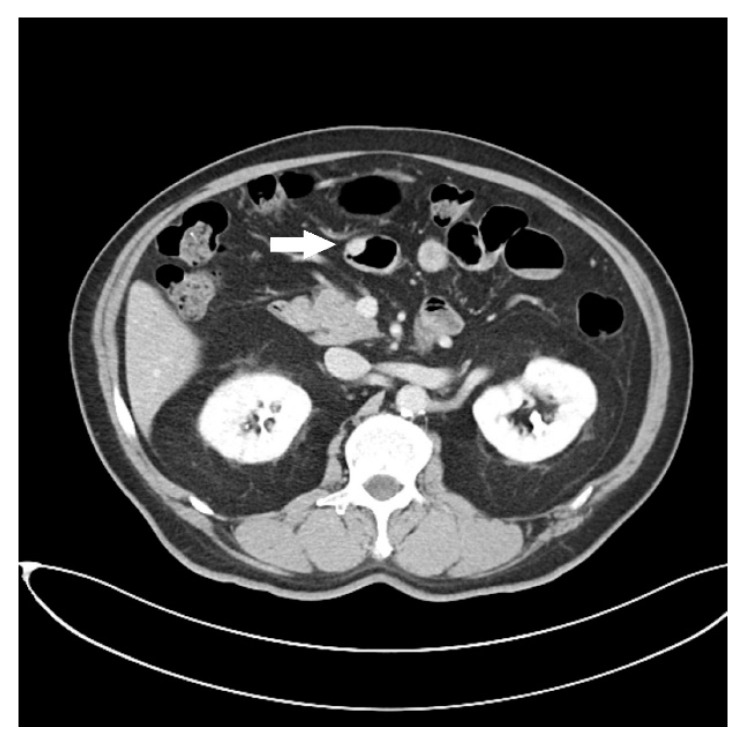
Abdominal CT finding of a small enhanced nodule in the jejunum (arrow).
